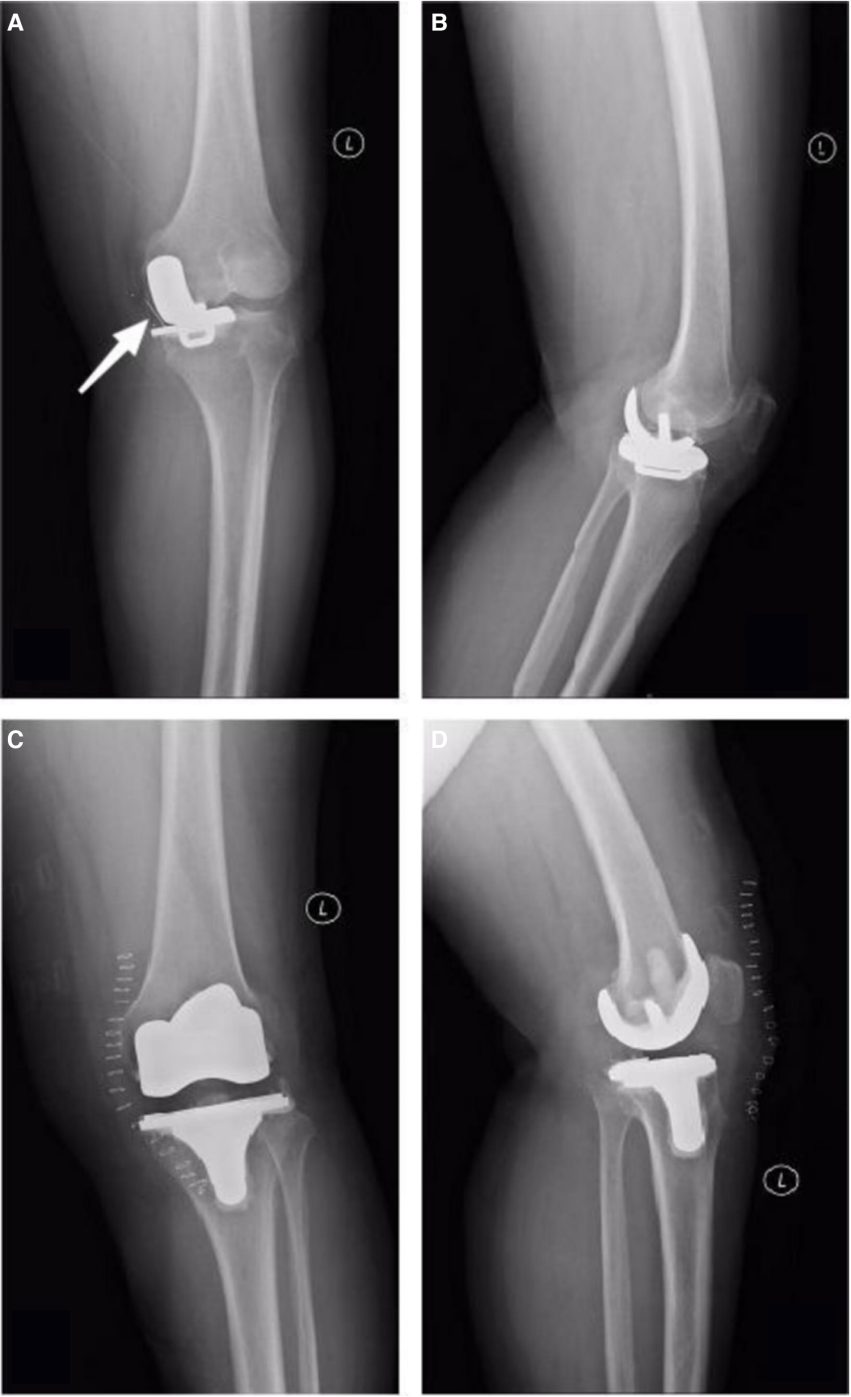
X-rays 5 months after UKA of the left knee and 3 days after conversion to TKA in a 65-year-old woman, White arrow: Poly backed out (A) Anteroposterior position of left knee joint 5 months after UKA, Poly backed out. (B) Lateral position of left knee joint 5 months after UKA. (C) The prosthesis of left knee joint was removed, and the surgical method was changed to TKA, anteroposterior position of left knee joint 3 days after TKA. (D) Lateral position of left knee joint 3 days after TKA.
